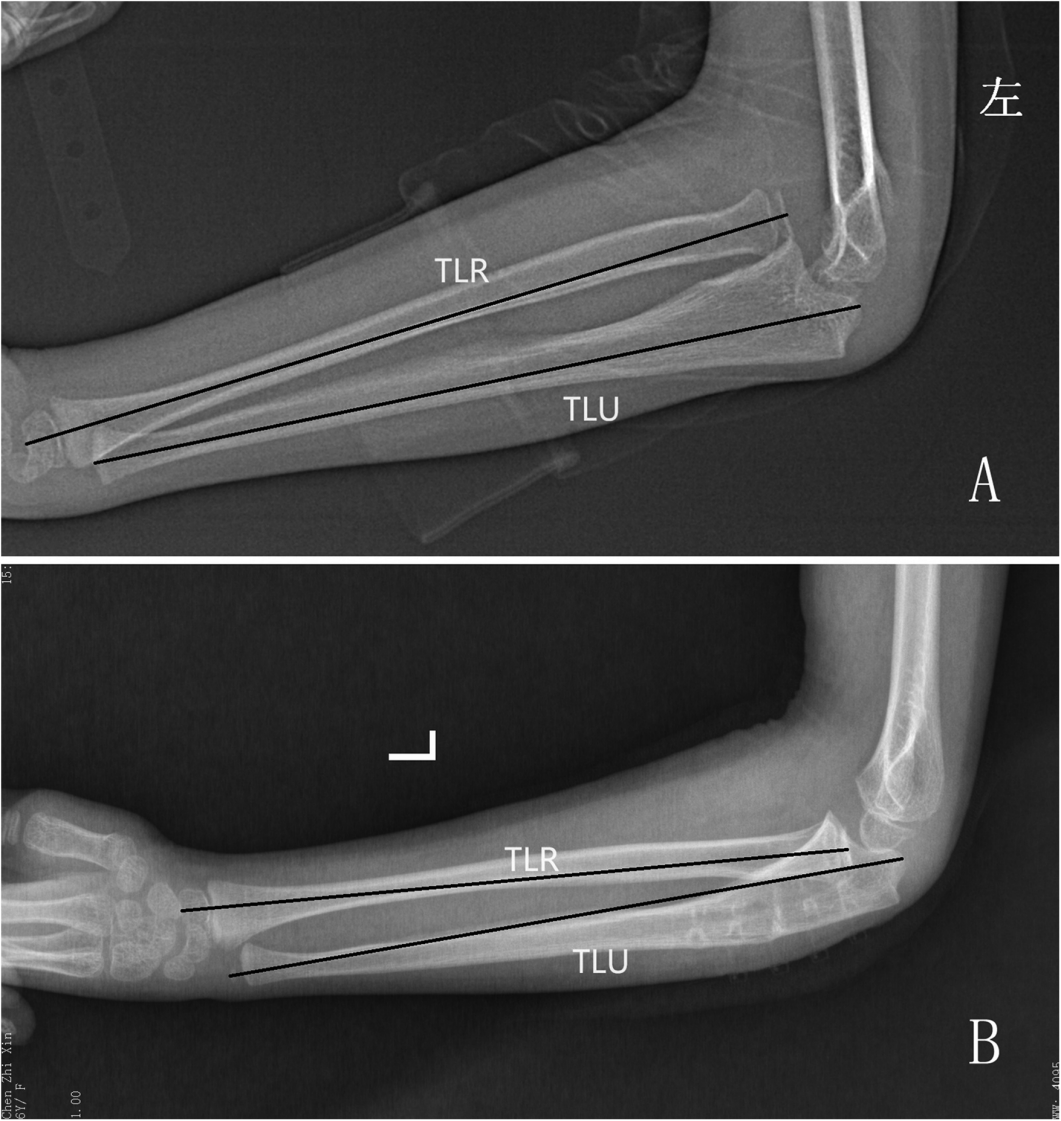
X-rays of neglected Monteggia fracture children, length ratio between the ulna and radius improved after operation. (A) Pre-operation, (B) Post-operation, TLU, total length of ulna; TLR, total length of radial.
